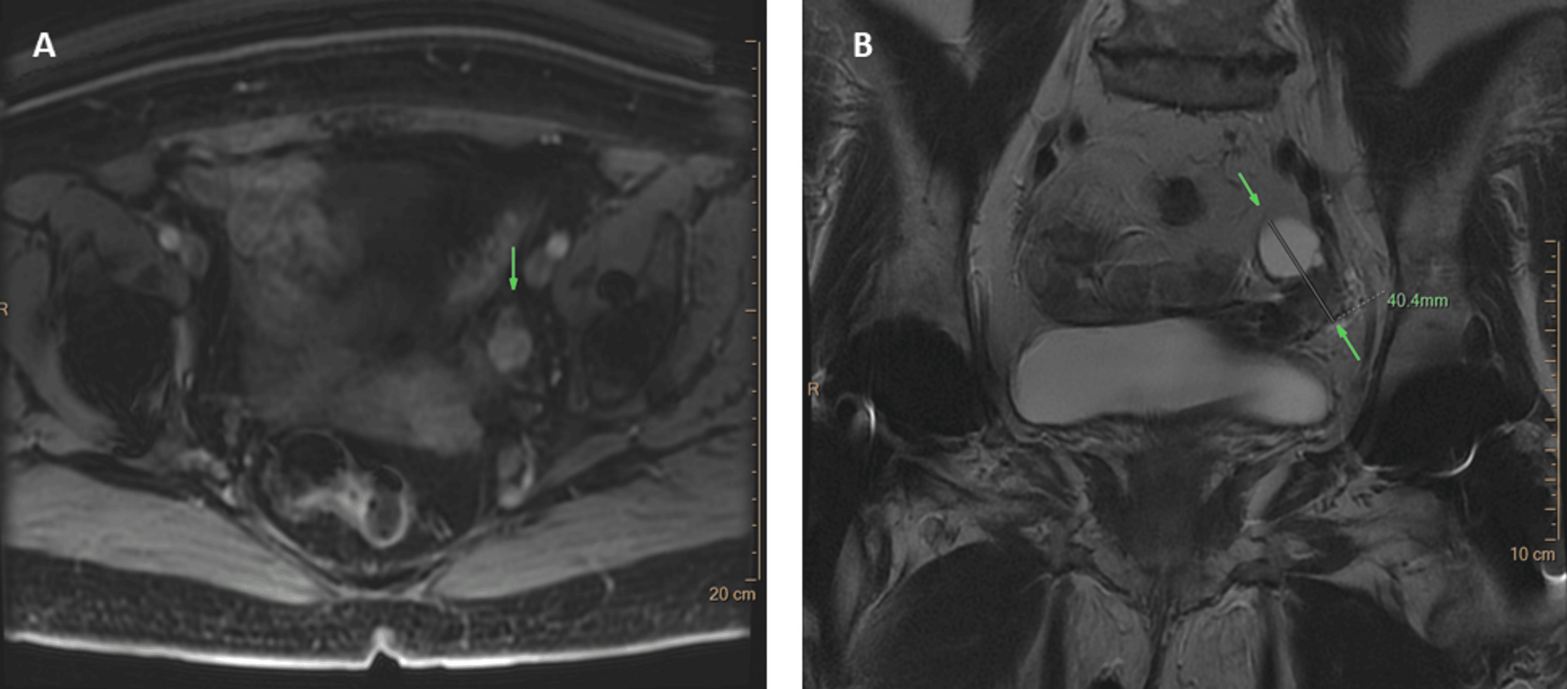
Pelvic MRI of Case 1 Left (panel A): Axial T1-weighted LAVA 90 s after IV Gd injection. The solid compartment shows enhancement (green arrow). Right (panel B): Coronal T2-weighted image. The left ovary shows a part with high signal intensity (SI) representing the cystic component (upper arrow) with adjacent microcysts and a part with low SI representing the solid compartment of the lesion (lower arrow).
